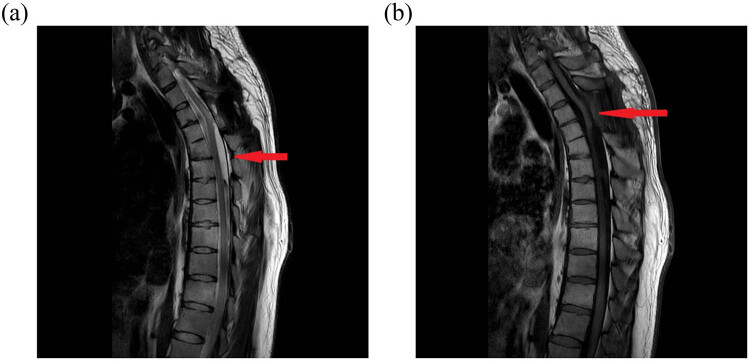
(a) T2 images from a sagittal plain MRI scan re-examined 6 months following surgery. The cystic space that was occupied vanished without a trace. When compared to before surgery, the spinal cord morphology is dramatically improved. (b) T1 images of sagittal plain MRI scan re-examined 6 months following surgery. Cystic space that was occupied vanished without recurrence. When compared to before surgery, the spinal cord morphology is dramatically improved.
